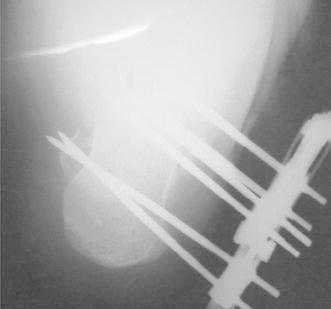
Postoperative radiograph on axial view
